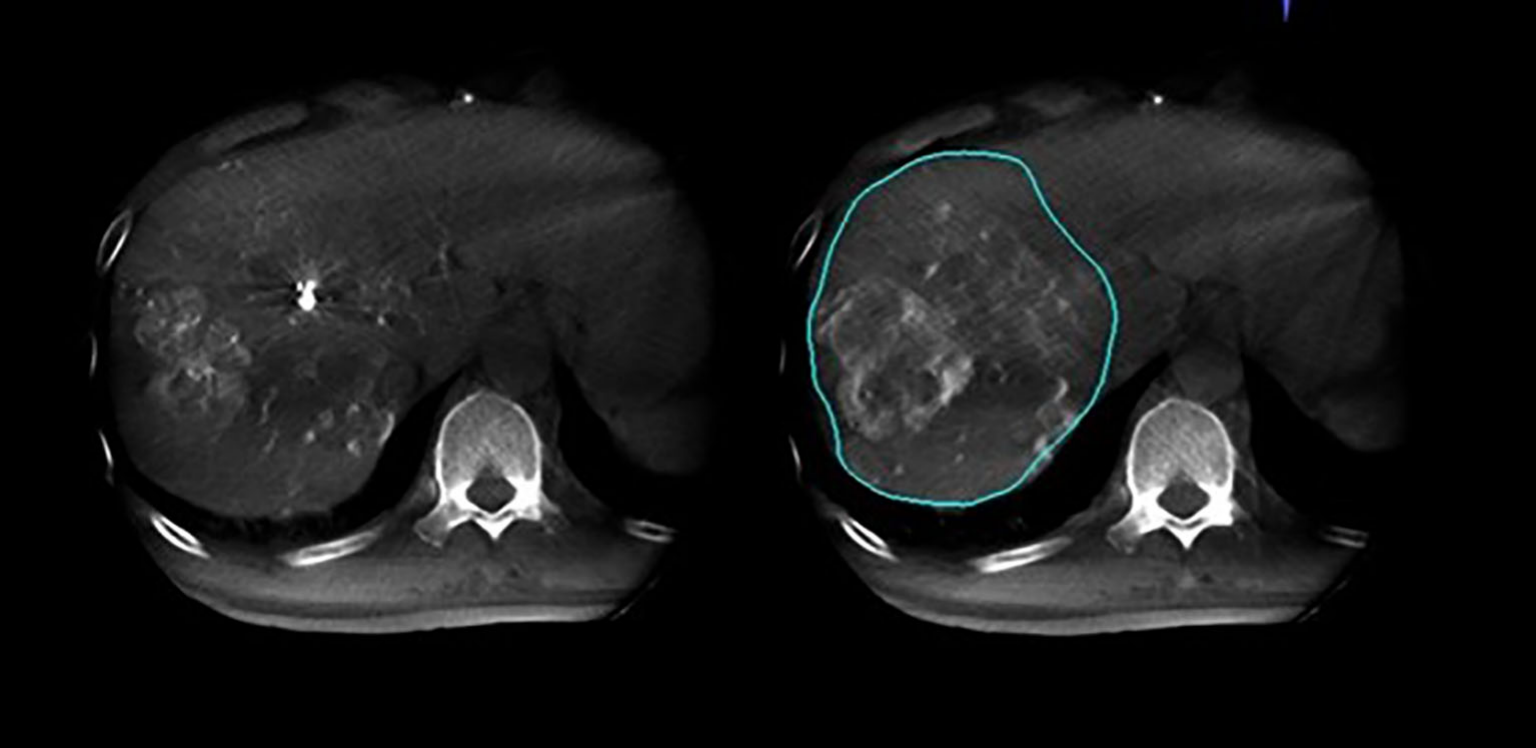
Arterial phase and delayed phase contrast-enhanced dual CBCT images acquired after injection into the segmental right hepatic lobe. The perfusion area can be assessed based on the delayed phase image on the right.
